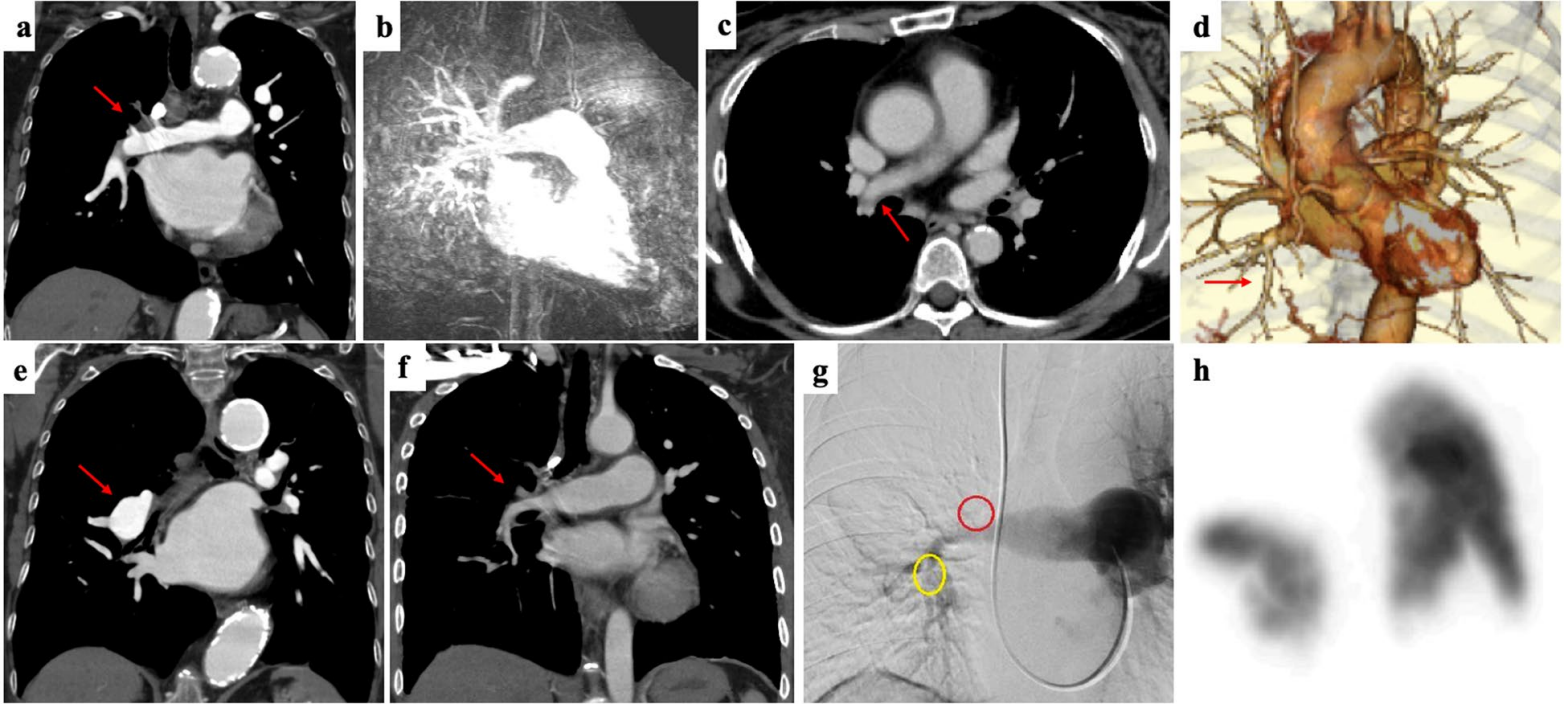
Representative pulmonary artery involvement images. Occlusion of the right upper pulmonary artery branches on enhanced computed tomography (CT) (a). Occlusion of the left main pulmonary artery on magnetic resonance angiography (b). The right main pulmonary artery vessel wall thickness (c) and stenosis of the lower pulmonary artery branches (d) on enhanced CT. Dilation of the right main pulmonary artery (e). Occlusion of the right pulmonary artery branches on enhanced CT (f) and angiography (g). A perfusion defect in the right lung (h)
